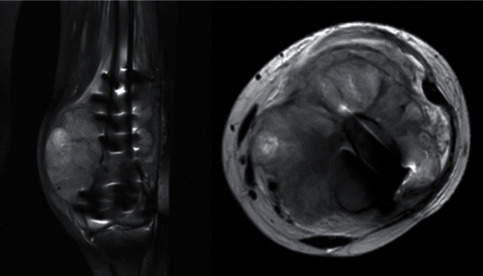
MRI scans of the bone mass that developed 3 months after operation.
